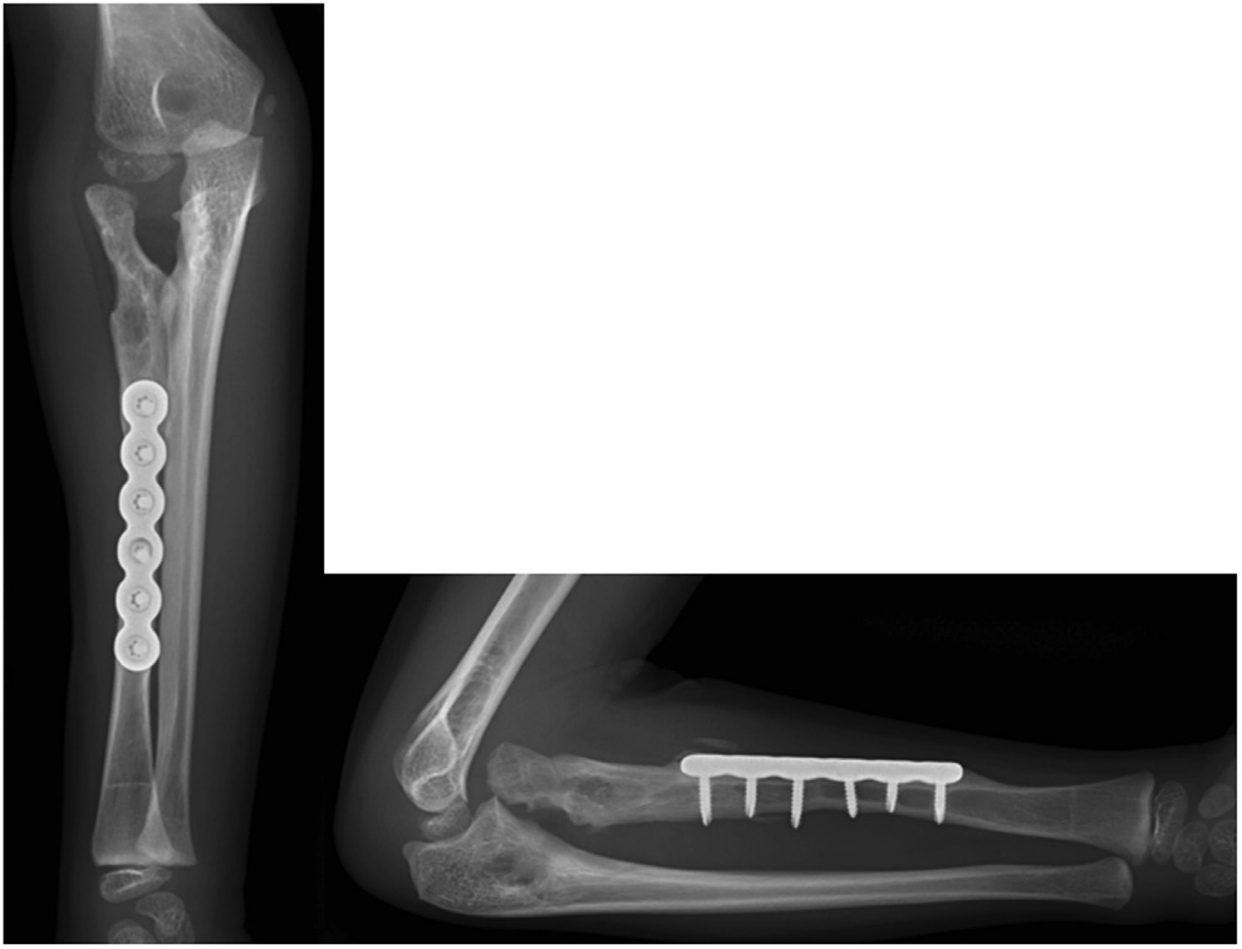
Radiographs 1 year postoperatively showing that the synostosis space remained separated but the radial head was anteriorly dislocated.
